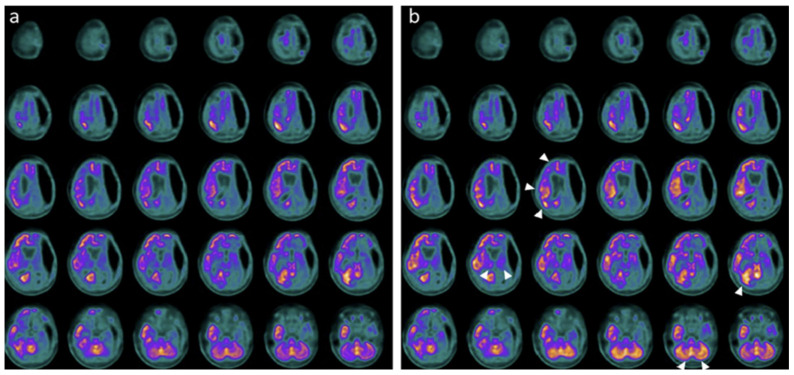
PET before and after the treatment with hr-NGF in the third TBI patient. (a,b) Brain 18F-FDG PET axial slices performed before (a) and after (b) hr-NGF treatment. A severe reduction in 18F-FDG uptake was observed in all cortical and subcortical regions of the left hemisphere, whereas a mild reduction was detected in the whole cerebellum and all cortical and subcortical regions of the right hemisphere (a). After hr-NGF administration, an increase in radiotracer uptake was found in the right frontal cortex (+11%), right temporal cortex (+15%), right parietal cortex (+14%), right occipital cortex (+22%), right and left thalamus (+10% and +7%, respectively), and cerebellum (+33%). The white arrows delineate the aforementioned areas. (b).
